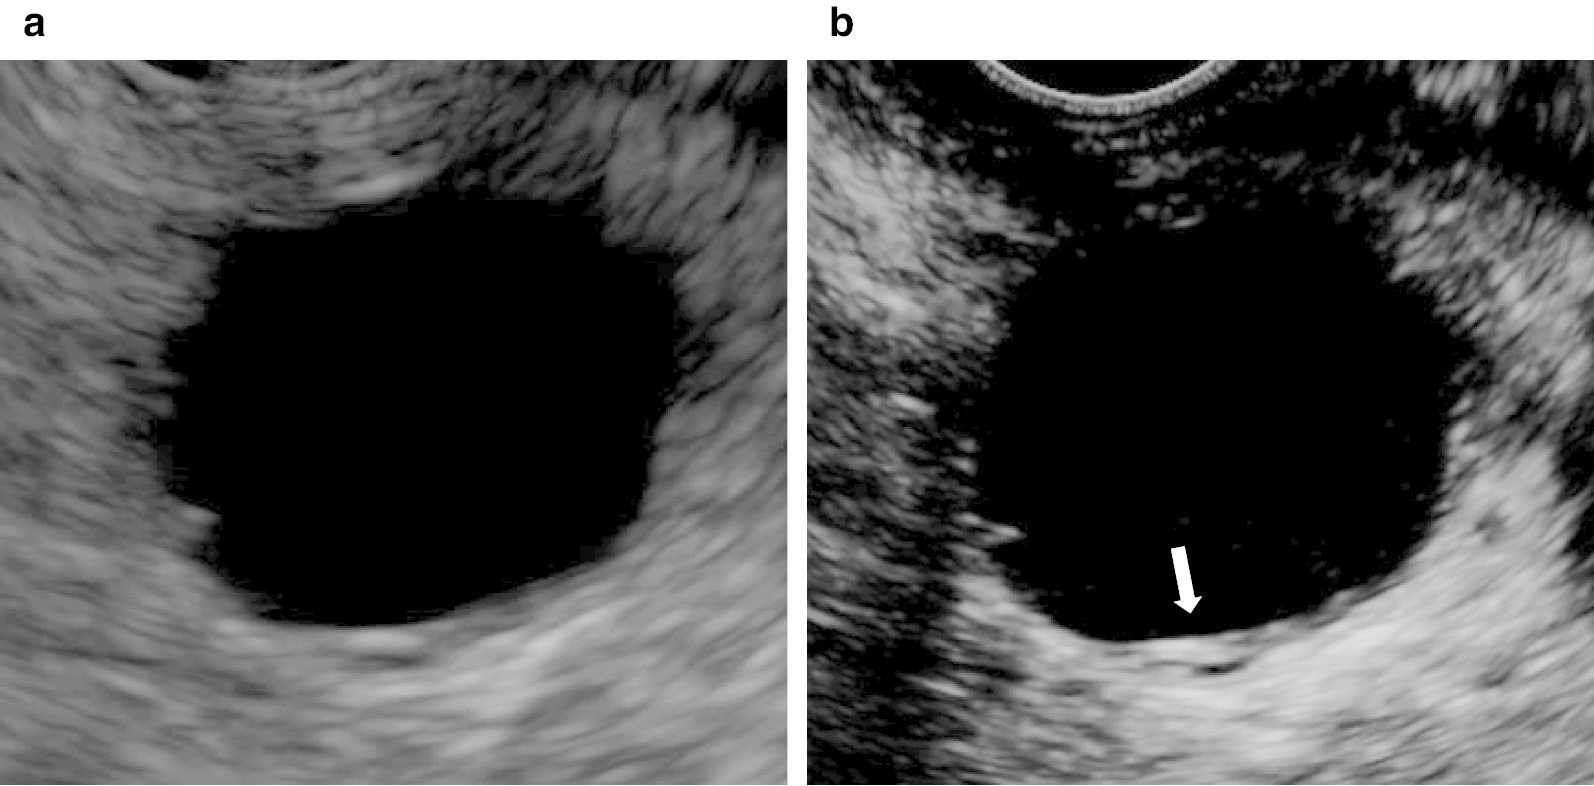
Endoscopic ultrasonography (EUS) showed a unilocular cystic lesion with a partial thickened wall in the pancreatic tail (a). The thickened wall was enhanced (arrow) when Sonazoid was administered (b)
